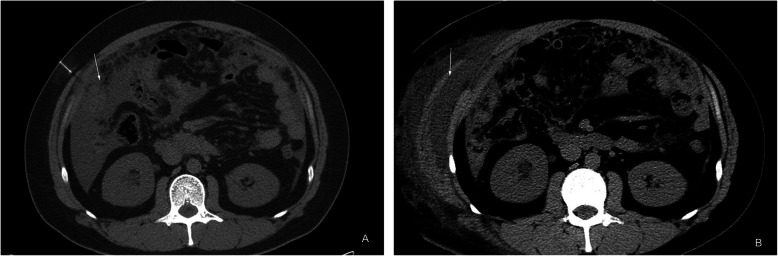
a, b One patient with tuberculosis. a Nodular peritoneal thickening; b Edema of the abdominal wall soft tissues by CT scan upon re-examination
